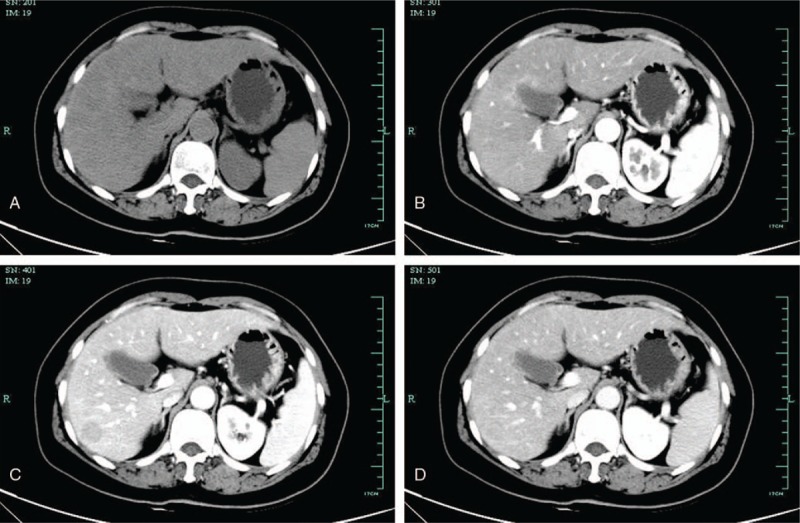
Liver contrast-enhanced computed tomography findings. There was a circular and low-density lesion in the right posterior lobe of the liver, approximately 22.0 × 18.7 mm in size. The lesion showed relatively low density in arterial phase and faded signal in portal phase and delayed phase. (A: plain scanning phase; B: arterial phase; C: portal phase; D: delayed phase).
